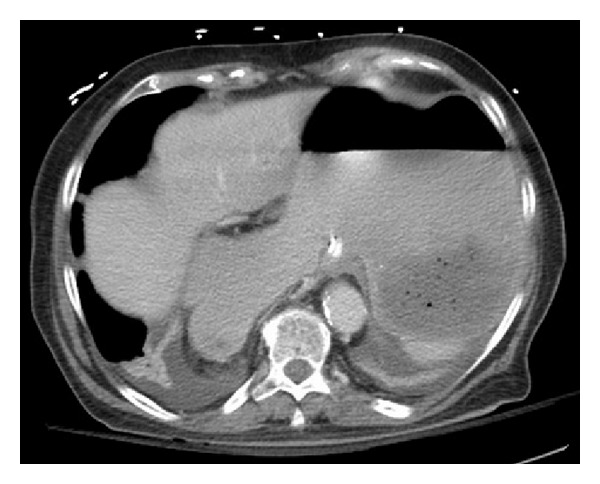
Transverse CT showing severe stomach distension.
